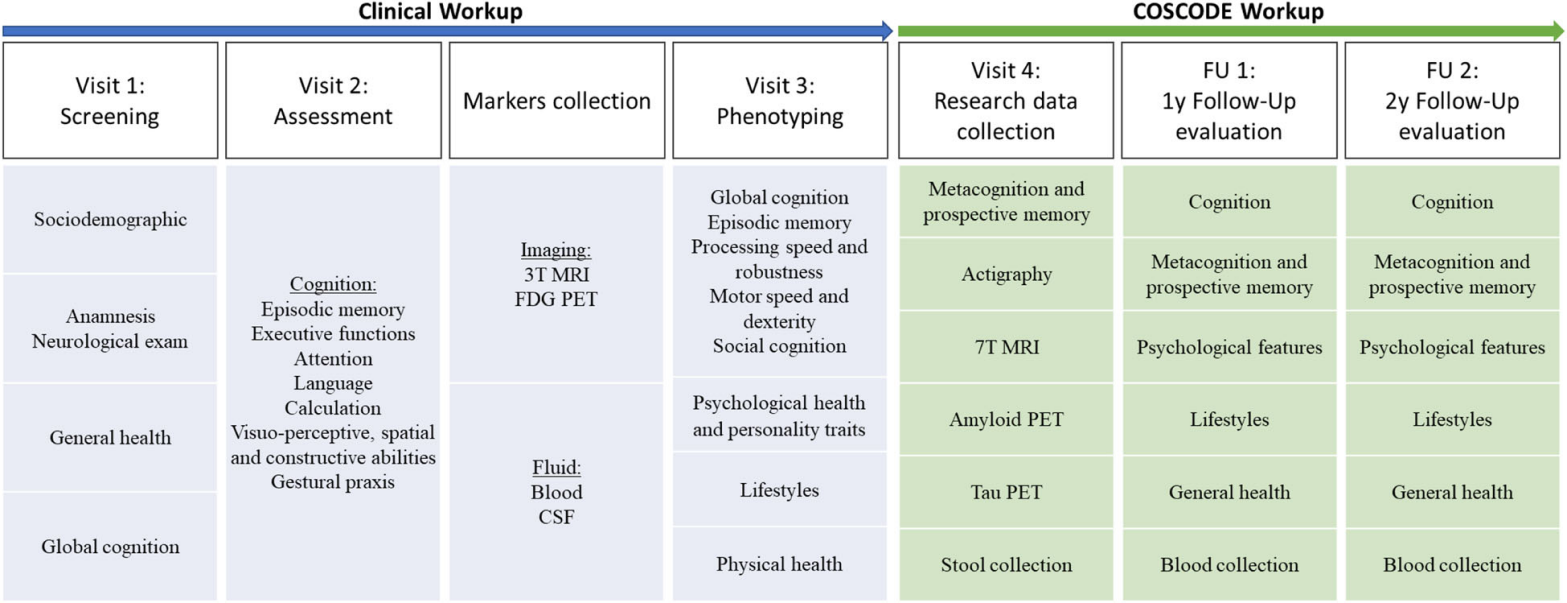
Clinical and research workups. The figure shows the clinical workup of the GMC and the research workup of COSCODE. During the first visit, sociodemographic features, general health, and global cognition (MMSE, Clock test, Three-Objects-Three-Places) are evaluated. In this visit, medical, family history, and medication information are collected, and a neurological exam is performed. Visit 2 consists of a complete neuropsychological assessment. Visit 3 includes scales and questionnaires on psychological health, lifestyles, and some additional cognitive tests. After Visit 3, the individual comes back to the GMC for a diagnostic restitution with a physician and a neuropsychologist. In this session, the COSCODE project will be proposed. During the COSCODE workup, the metacognition task, scales, and questionnaires on metacognition will be administered. Stool and saliva will be collected for all participants. 60 participants will undergo 7T MRI. Amyloid and tau PET, as well as actigraphy will be performed on around 60% of the sample. Behavioral follow-up at 1 and 2 years will be done for all participants
